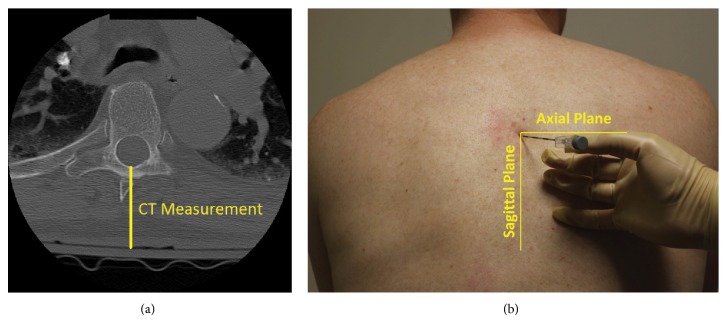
(a) Axial computed tomography (CT) image of thorax with demonstrated measurement technique; (b) demonstration of appropriate planes with epidural needle placed on back.
